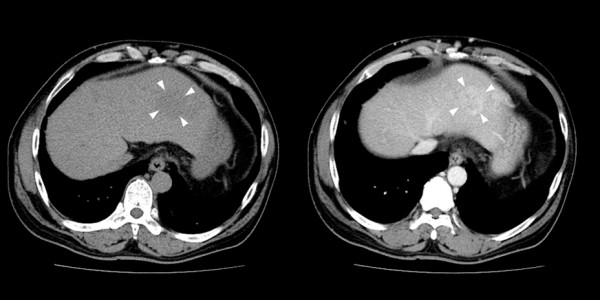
The abdominal computed tomograph in a patient of hepatic angiosarcoma (Case 9). A low-density mass (3.5 cm) with persistent contrast enhancement (29/54/68 hu) in left hepatic lobe is noted (arrowhead) (left: non-contrast phase, right: venous phase). Several enhancing lesions (1-3 cm) in right lobe of liver S5 are not well demonstrated in the venous phase (not shown in figure). Irregular liver surface, splenomegaly, and collateral circulation are noted, indicating cirrhosis.
